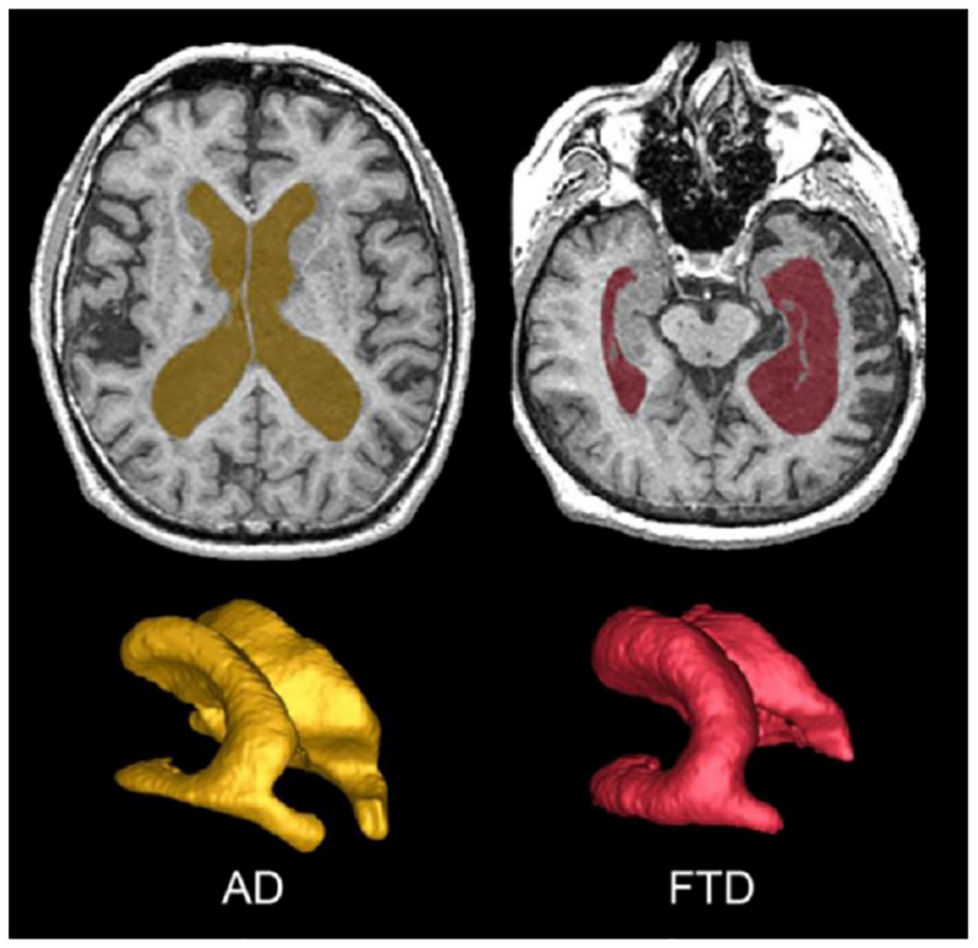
Top row shows axial view of vCSF segmentation overlayed on T1 MRI for patients with AD (left) and FTD (right). Bottom row shows 3D surface volume renderings of the vCSF segmentation. Note the differences in ventricle size and the hemispheric differences between the two neurodegenerative diseases.
